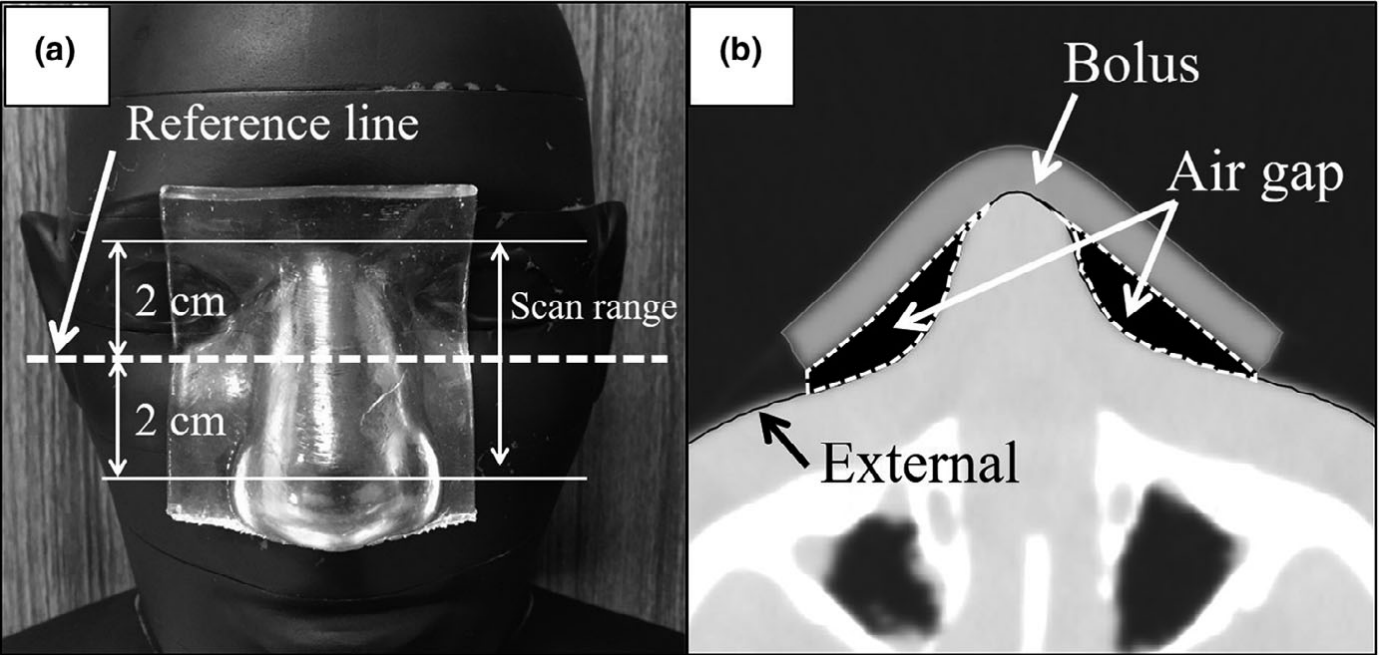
(a) Scanning range of the computed tomography image and (b) contour of the bolus and air gap.
